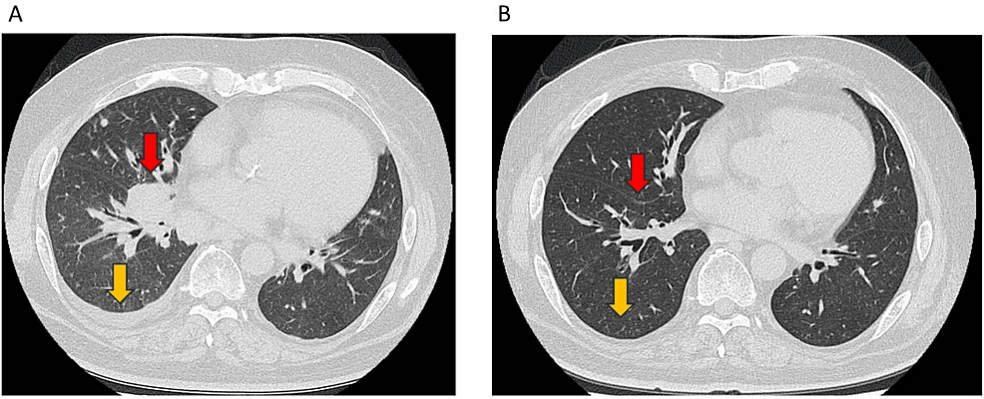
CT scan findings during the patient’s treatment (A) Computed tomography scan findings before first-line chemotherapy showing the right hilar tumor of approximately 4 cm in size (red arrow) and right pleural effusion (yellow arrow). (B) Both the right hilar tumor and right pleural effusion resolved after four courses of maintenance therapy.
